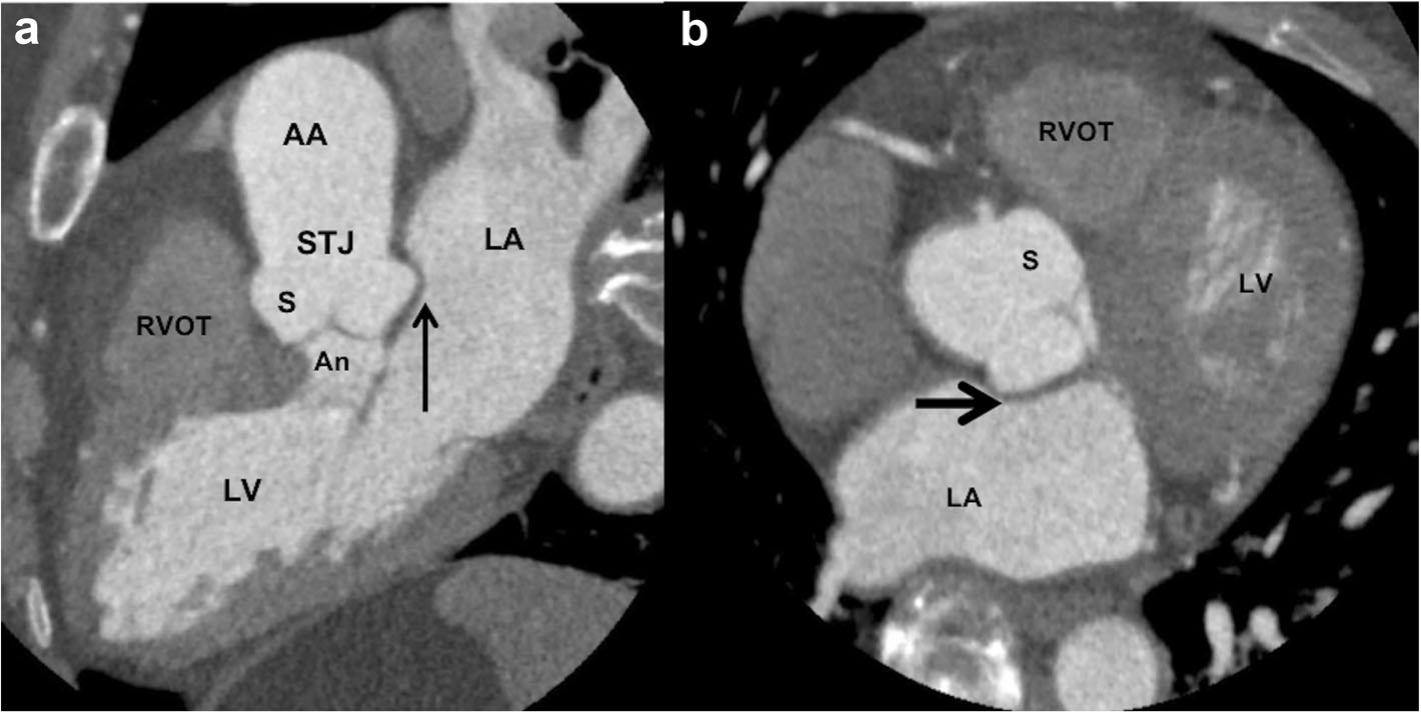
Reformatted 3-chamber view (a) and axial (b) CTA of the heart and proximal aorta showing the normal relation of the aortic root to the left atrium. No soft tissue should be present between the wall of the left coronary sinus and the left atrium (black arrow). In patients with aortic root infection, this space (black arrow) is increased with soft tissue. Abbreviations: AA, ascending aorta; LA, left atrium; LV, left ventricle; PA, pulmonary artery; An, annulus; S, sinus of Valsalva; STJ, sinotubular junction
